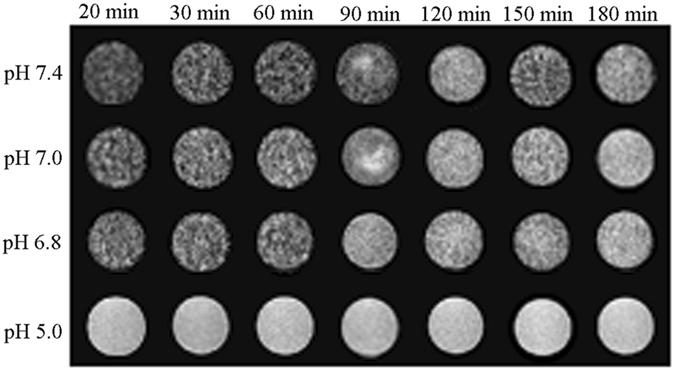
In vitro* MR images from NaGdF_4_@CaCO_3_-PEG at various pH (5.0, 6.8, 7.0 and 7.4) conditions along with the time.
